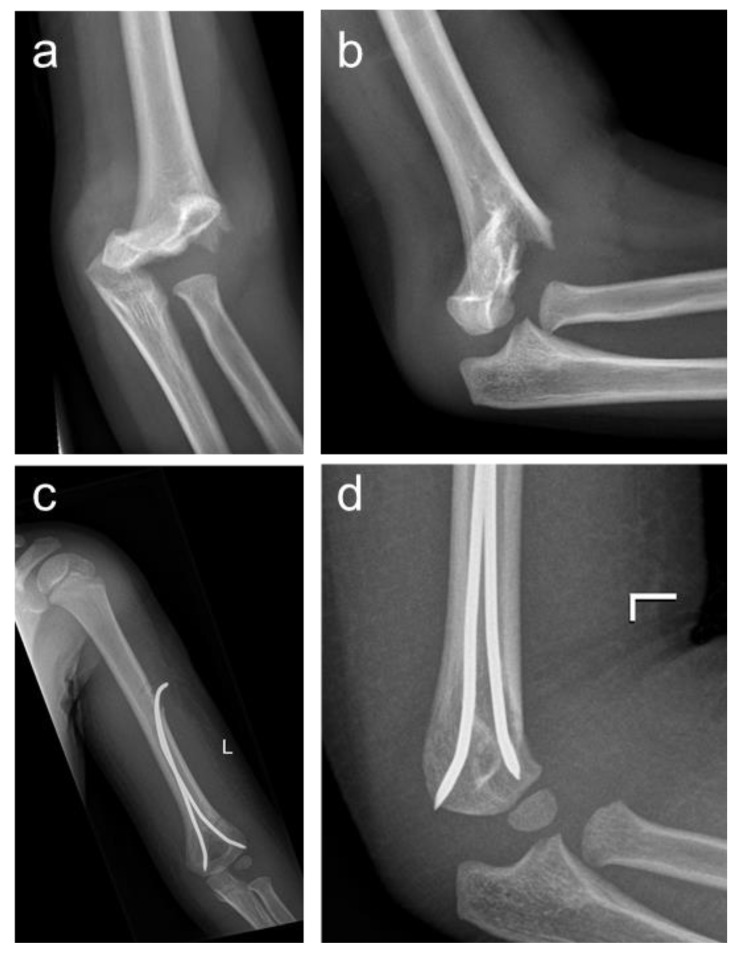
Anterior–posterior and lateral radiographs of a Gartland type 2 fracture (a,b); antegrade nailing was performed using 2 elastic nails (c,d).
